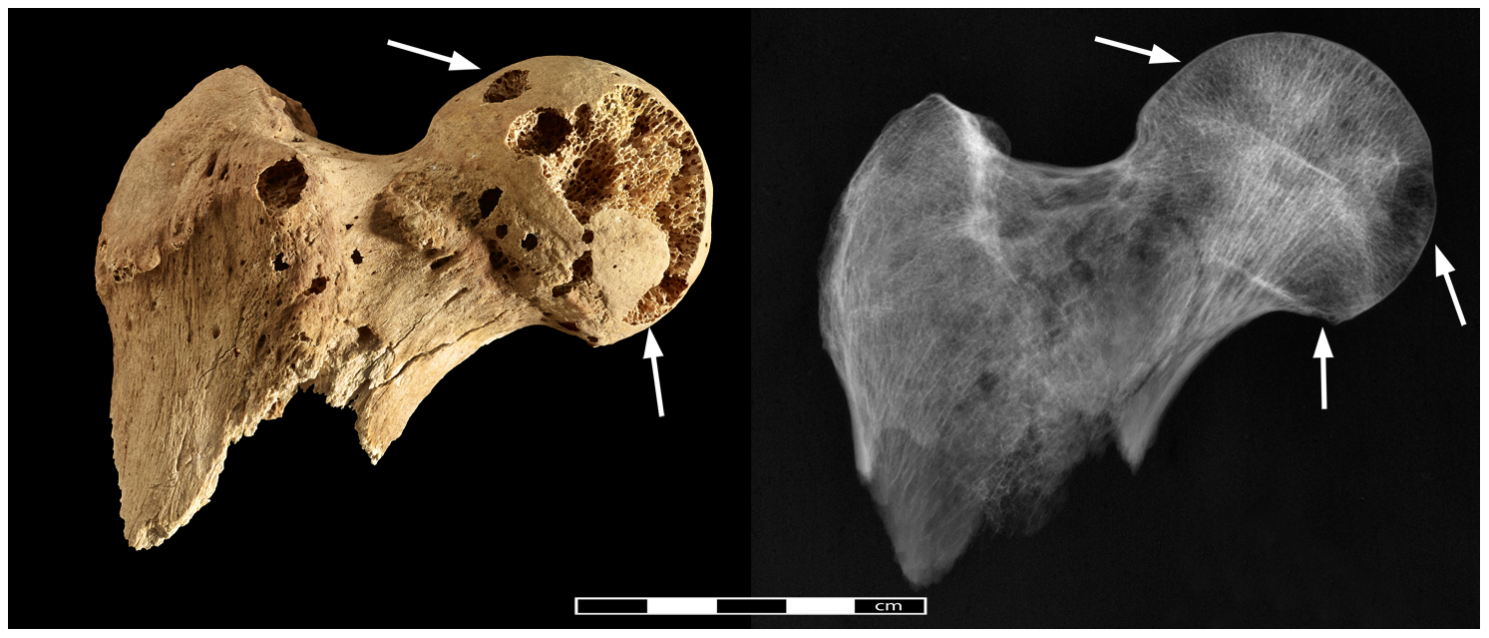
Pathological changes in the right femoral head. Photo- and radiograph of lytic lesions in the right femoral head (arrows indicate areas of pathological lesions).
